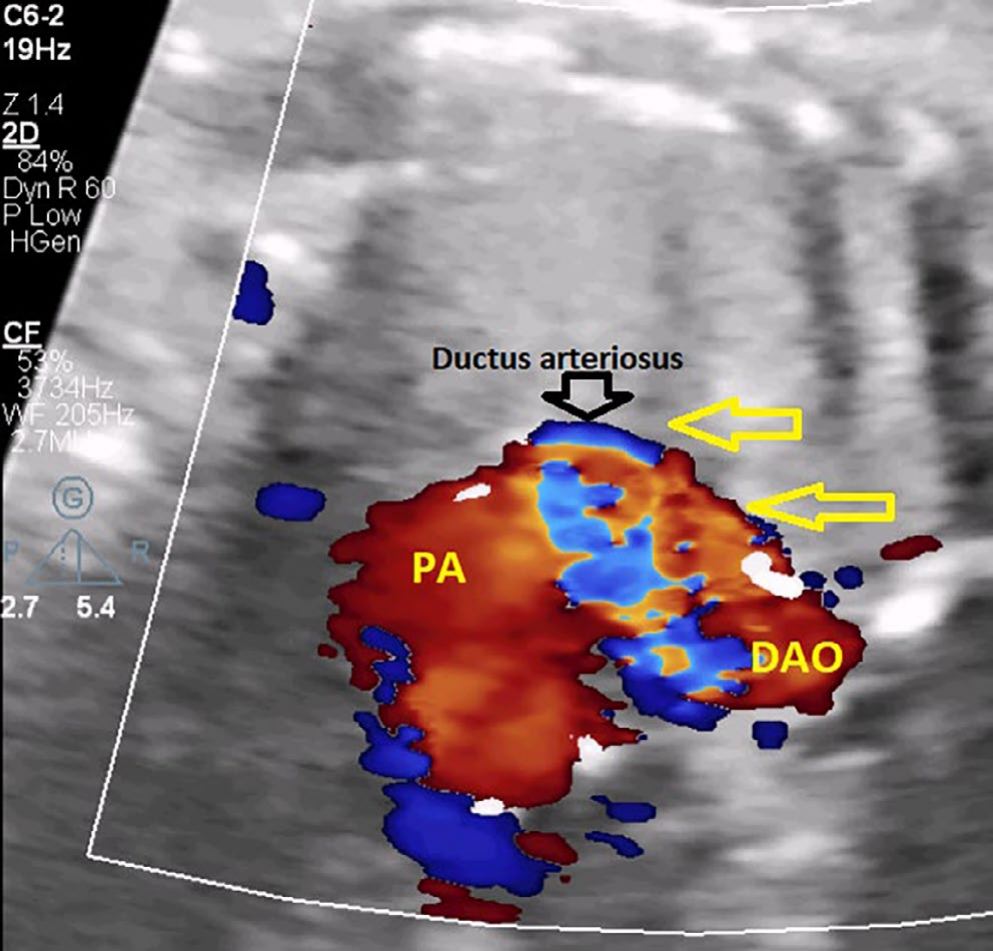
The four‐chamber view with color Doppler imaging demonstrates flow acceleration, evidenced by aliasing.
